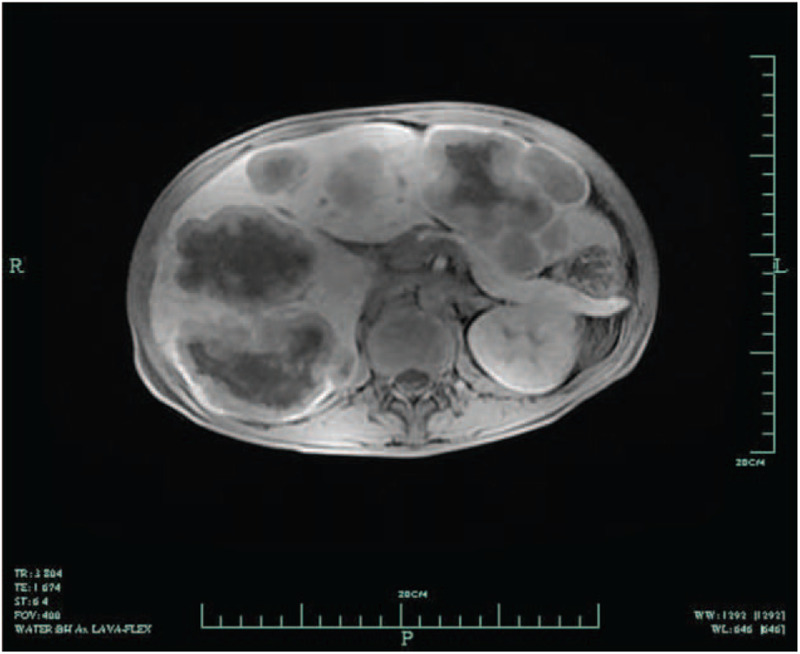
Liver magnetic resonance imaging. There were multiple intrahepatic lesions; abscesses and metastatic tumours were first considered. More enlarged lymph nodes were seen in the peritoneal and mesenterium areas.
